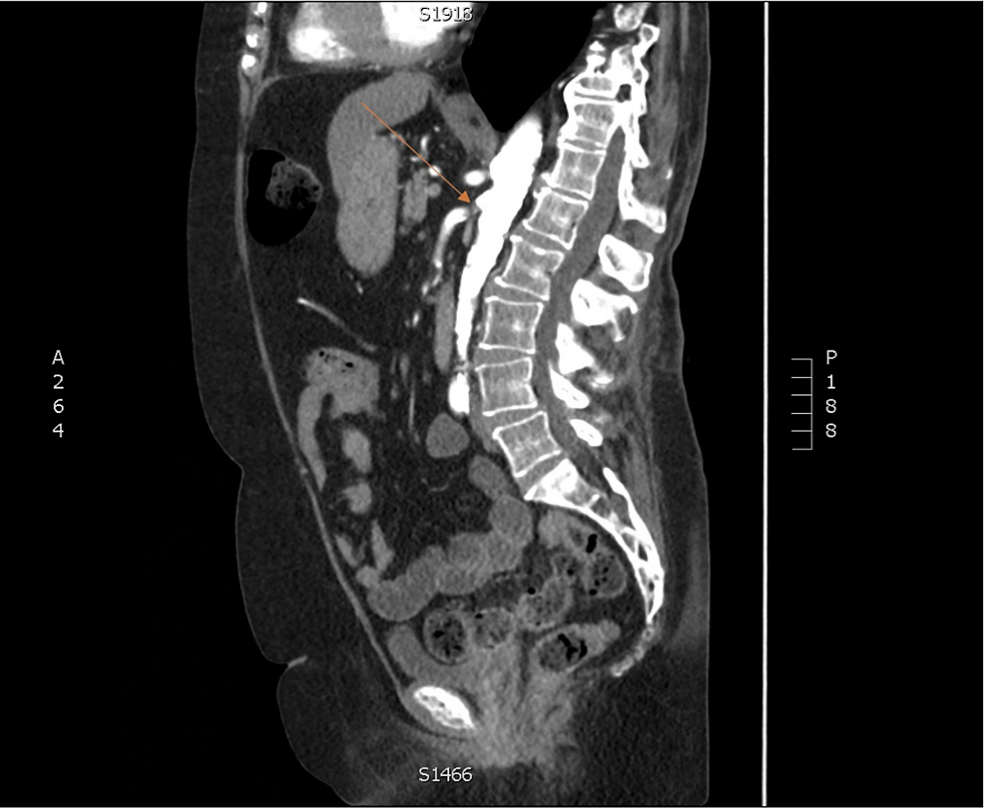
Sagittal CT angiogram of the abdomen and pelvis of the patient demonstrating the stenosis in the superior mesenteric artery (arrow). CT, computed tomography
